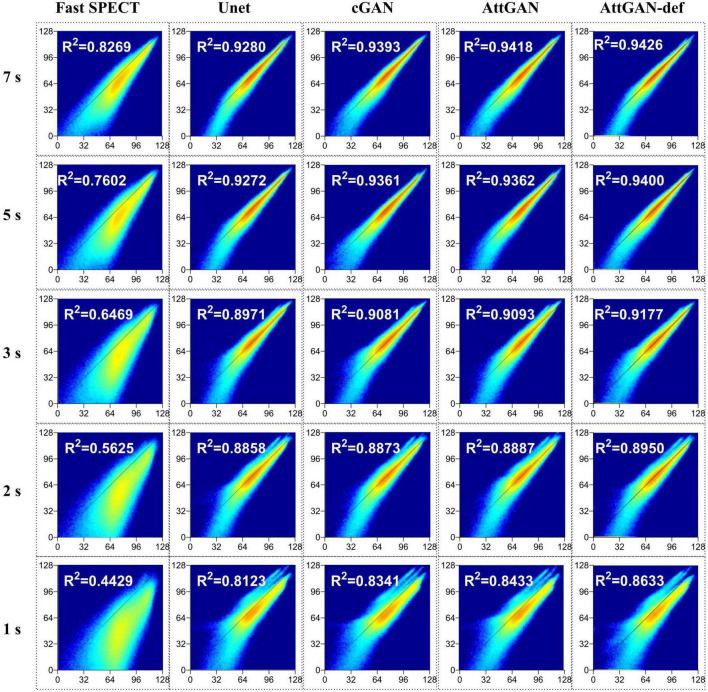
Joint histogram and linear regression analysis of fast SPECT and different denoised images in the 50 testing datasets for five shorter acquisition times. The filtered FT images are used as the reference.
